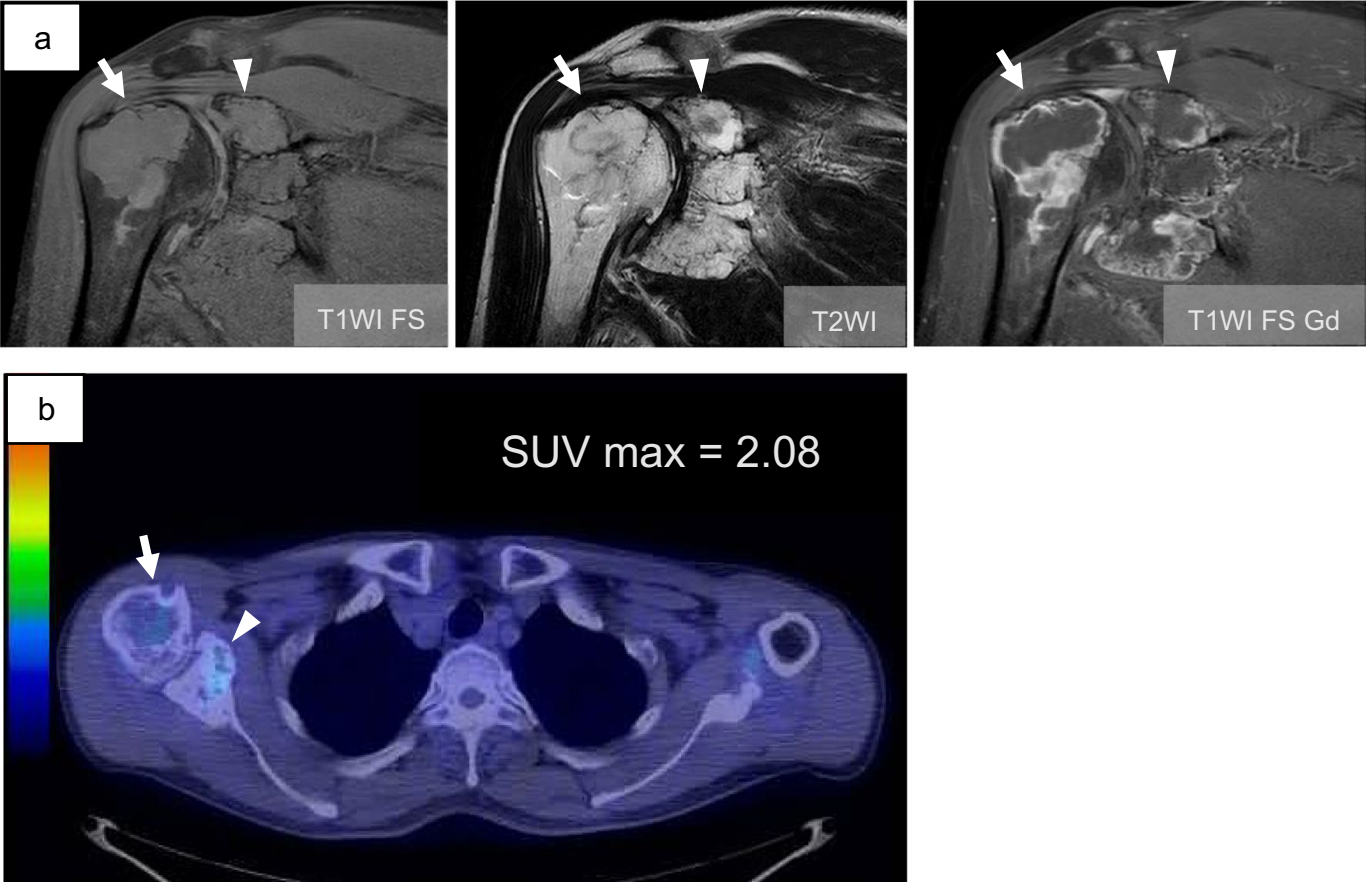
MRI and PET-CT image. a An MRI scan reveals the presence of multi-lobulated intramedullary tumors exhibiting iso-intensity on T1WI and homogeneous high signal intensity on T2WI with peripheral gadolinium enhancement in both the scapula (arrowhead) and proximal humerus (arrow). b PET-CT scan reveals SUV max value 2.08 hot spot in both scapula (arrowhead) and humeral head (arrow)
